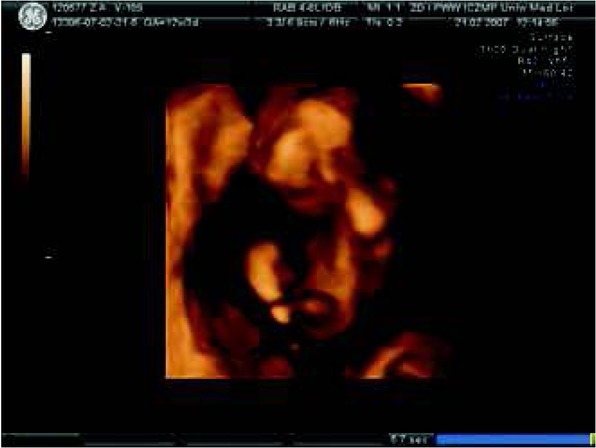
Fetal body in 3D ultrasound at the 12th week of gestation – one of the very first medical diagnostic imaging examinations. Ryc. 1. Zarysy całego płodu w badaniu USG w prezentacji 3D w 12 tyg. ciąży – jedno z pierwszych medycznych badań diagnostycznych.
